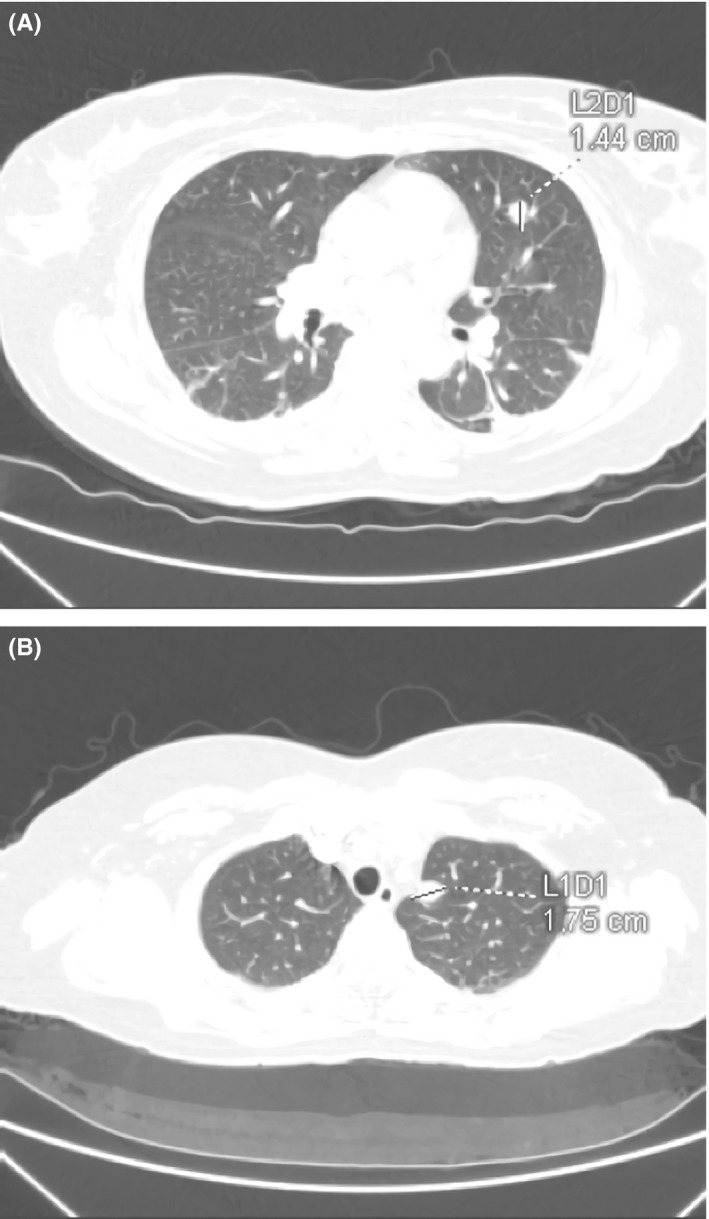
Thoracoabdominopelvic CT scan: Pulmonary nodules of 1.44 cm and 1.75 cm suggestive of pulmonary metastases
